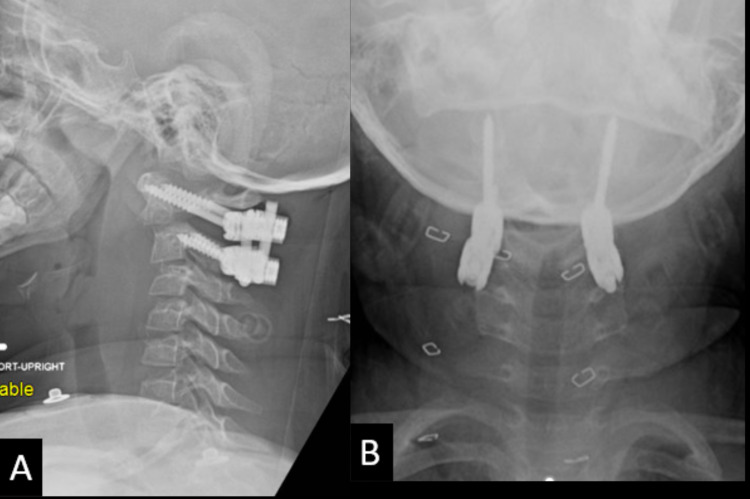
Lateral (A) and anterior-posterior (B) upright cervical spine plain films demonstrating placement of C1 lateral mass screws and C2 pars screws with improved fracture alignment and appropriate hardware placement.
